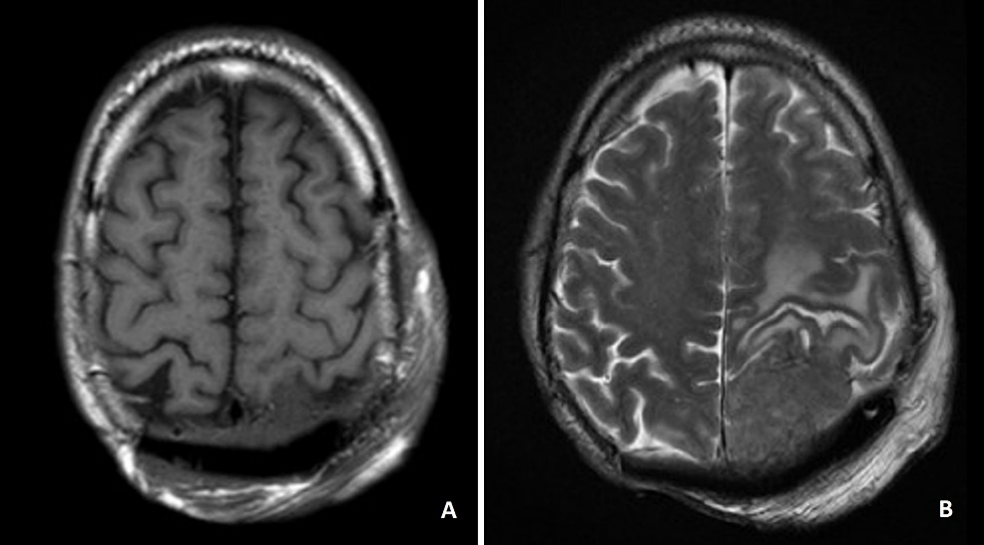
A - Brain MRI (axial, T2); parietal lesion compressing the brain parenchyma; B - Brain MRI (axial, T2); disease progression on chemotherapy
